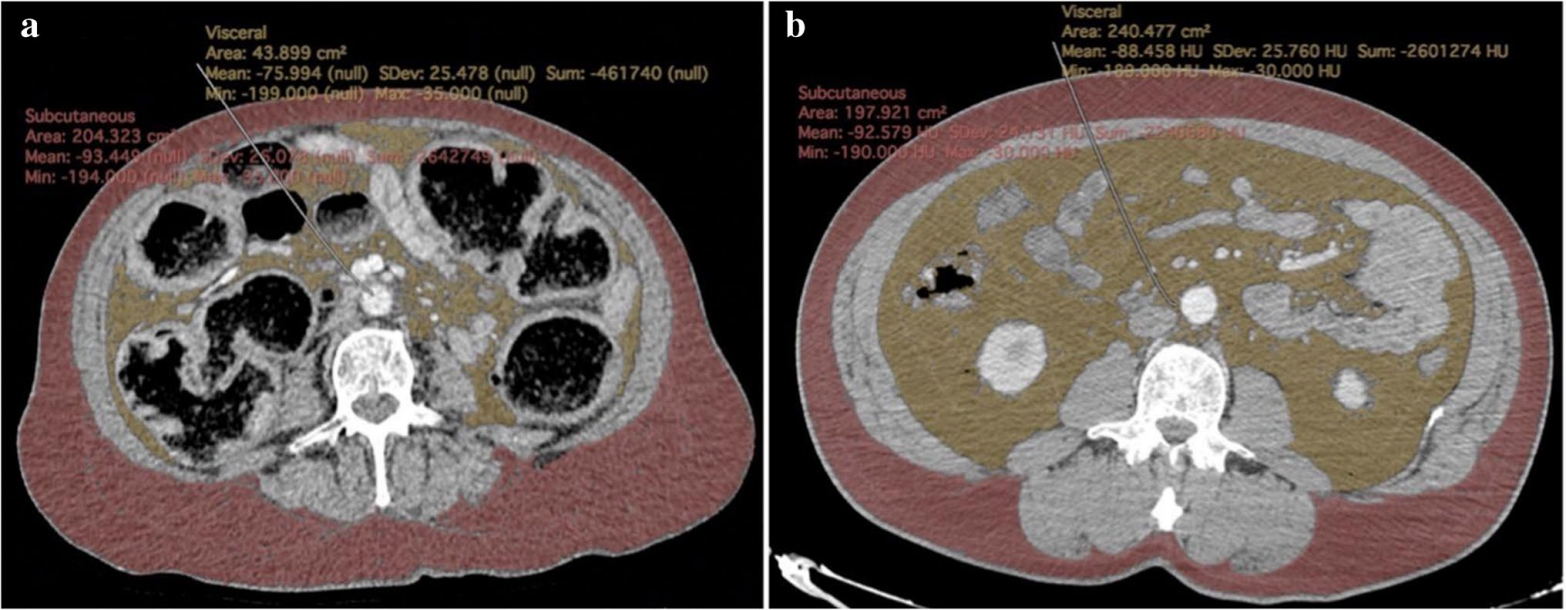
a Measurement of subcutaneous and visceral fat areas in axial computed tomography sections obtained at the L3-L4 vertebral level in a case of complicated diverticulitis presented with obstruction. b Measurement of subcutaneous and visceral fat area in axial computed tomography sections obtained from the L3-L4 vertebral level in a case of uncomplicated diverticulitis
